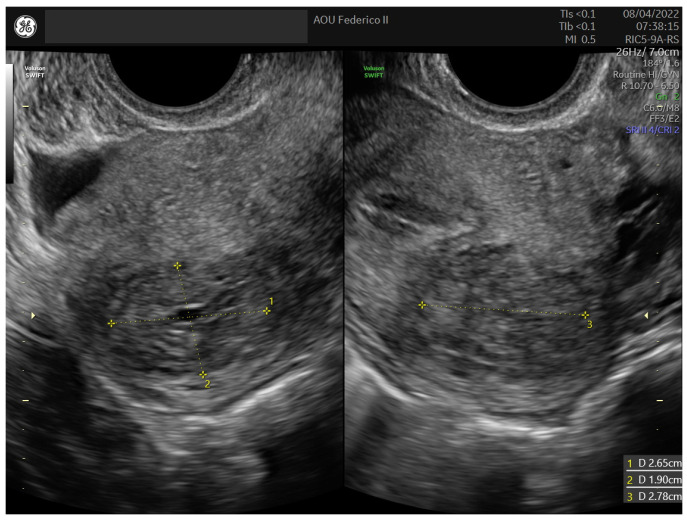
Sonographic appearance and measurements in three dimensions of the UTROSCT in Case 1.
